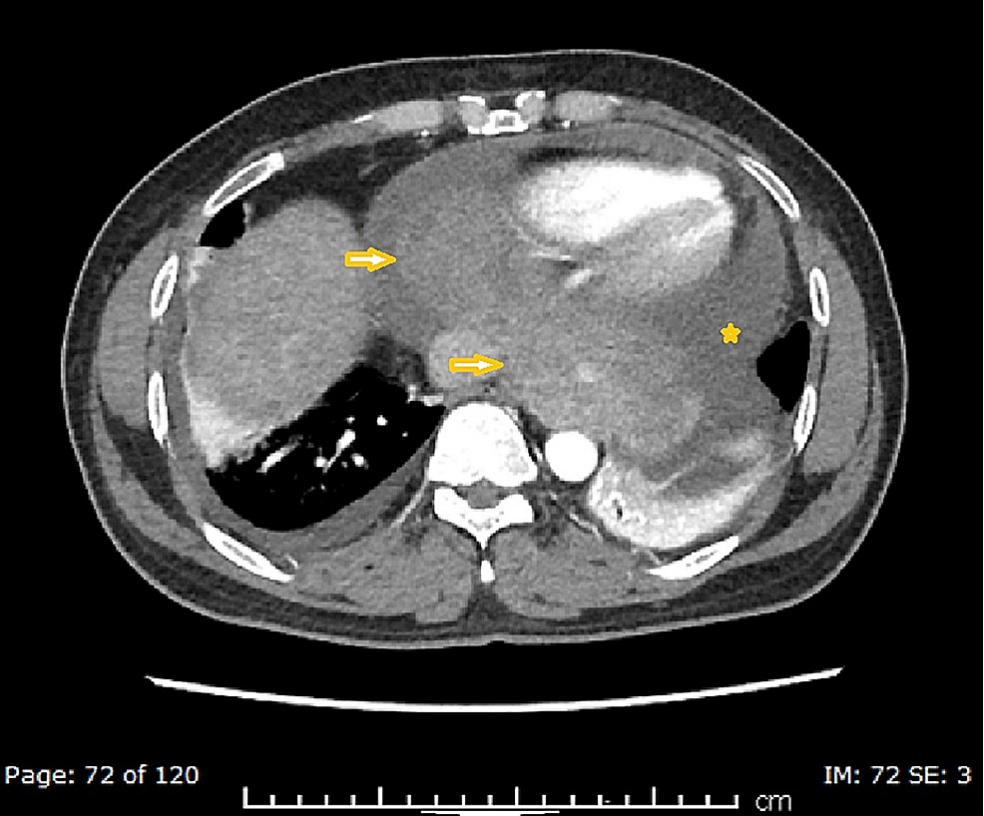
Axial contrast CT (arterial phase) images showing a large, heterogeneously enhancing pericardial mass (arrowheads) with pericardial effusion (star)
